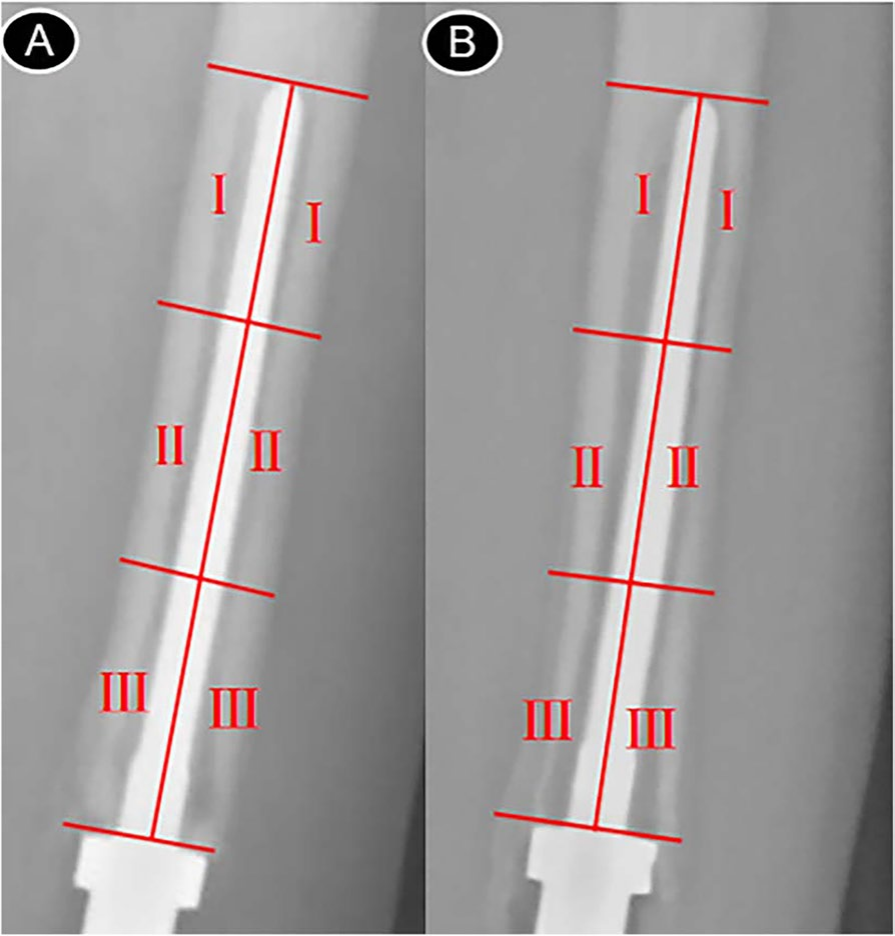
A and B are X-rays of the same patient at different times. A shows partition of the intramedullary stem after underwent tumor prostheses replacement. B shows partition of the intramedullary stem before revision surgery
